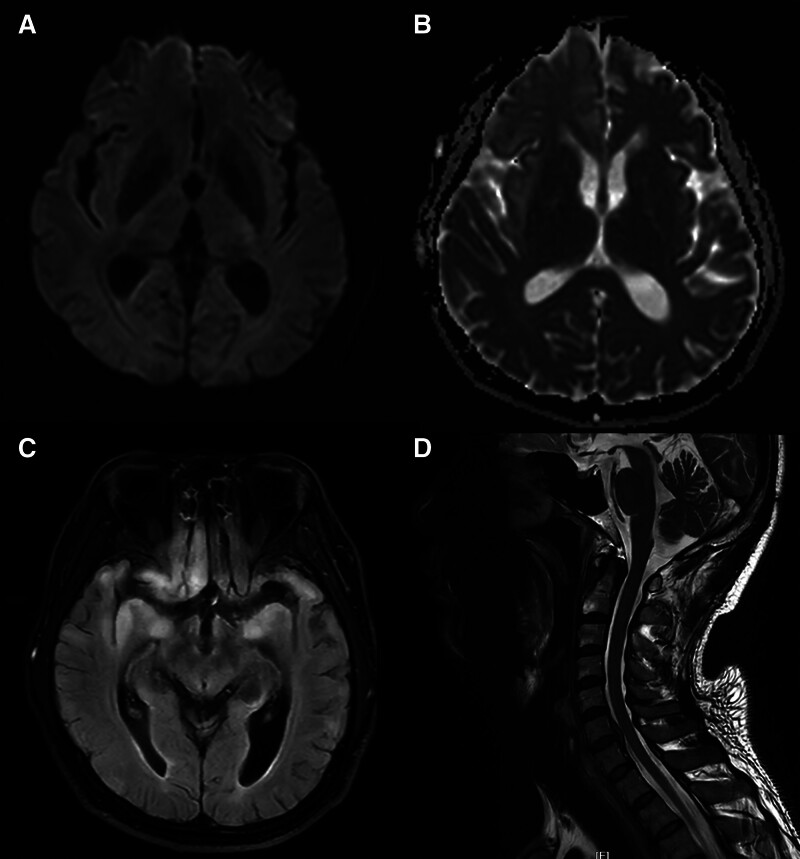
Head MRI findings on the thirteenth day of illness. The patient’s head DWI (A) and ADC (B) results were not abnormal. There is an increase in the number of flaky abnormal signal shadows seen on the T2 flair (C). MRI images of the cervical spine showed no abnormalities (D). ADC = apparent diffusion coefficient, DWI = diffusion-weighted imaging, MRI = magnetic resource imaging.
